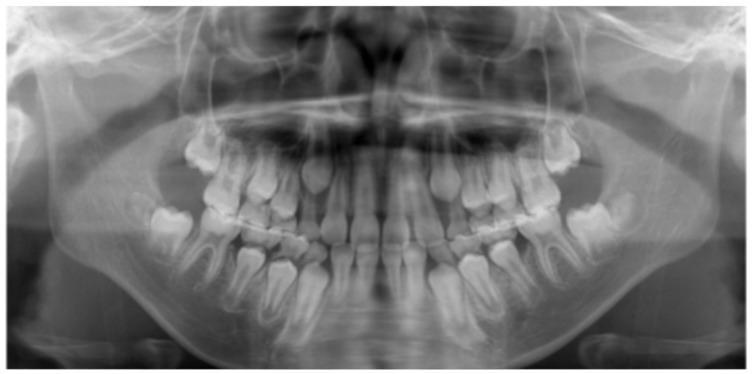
Panoramic radiography findings (age 9 years 11 months).
